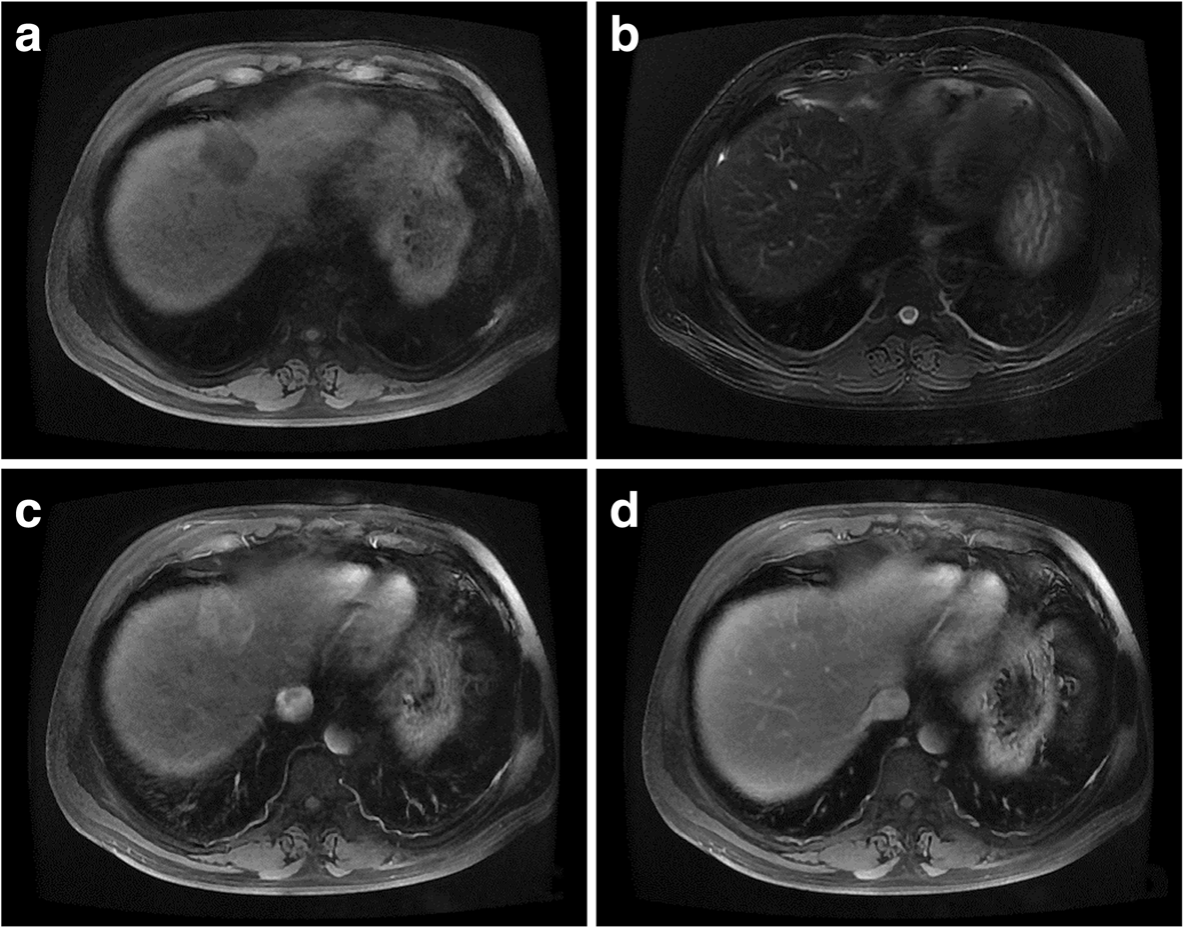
Magnetic resonance imaging scan of intrahepatic splenosis. Note a slightly hypointense mass on both T1-weighted (a) and T2-weighted (b) images. After the injection of gadoxetic acid, the mass became strongly heterogeneous and hyperintense during the arterial phase (c) and relatively hypointense during the portal phase (d)
